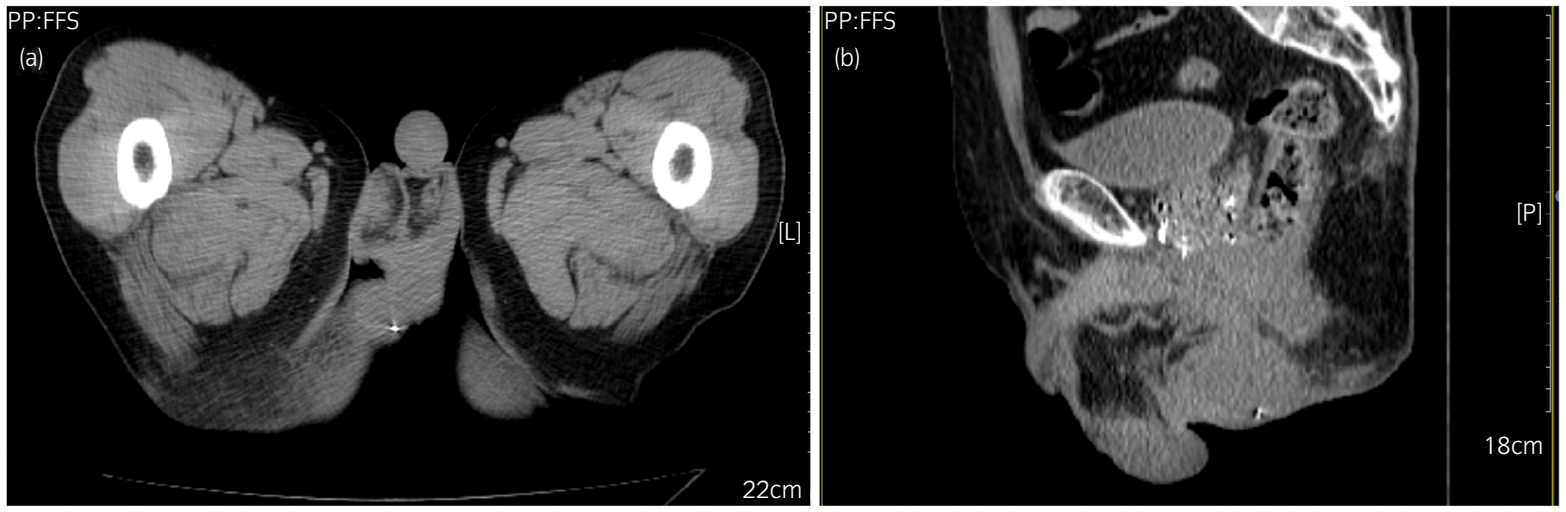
Abdominal computed tomography showed high density area suggestive of a hydrogel seed on the right side of the perineum––horizontal (a) and sagittal (b) views.
